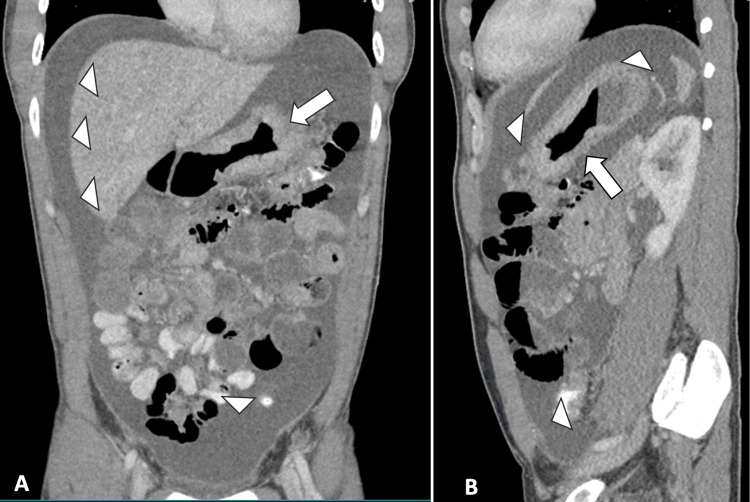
CT scan of the abdomen and pelvis with oral and intravenous contrast. (A) Coronal and (B) sagittal views showing gastric thickening (arrow) and diffuse ascites (arrowheads). CT: computed tomography.
